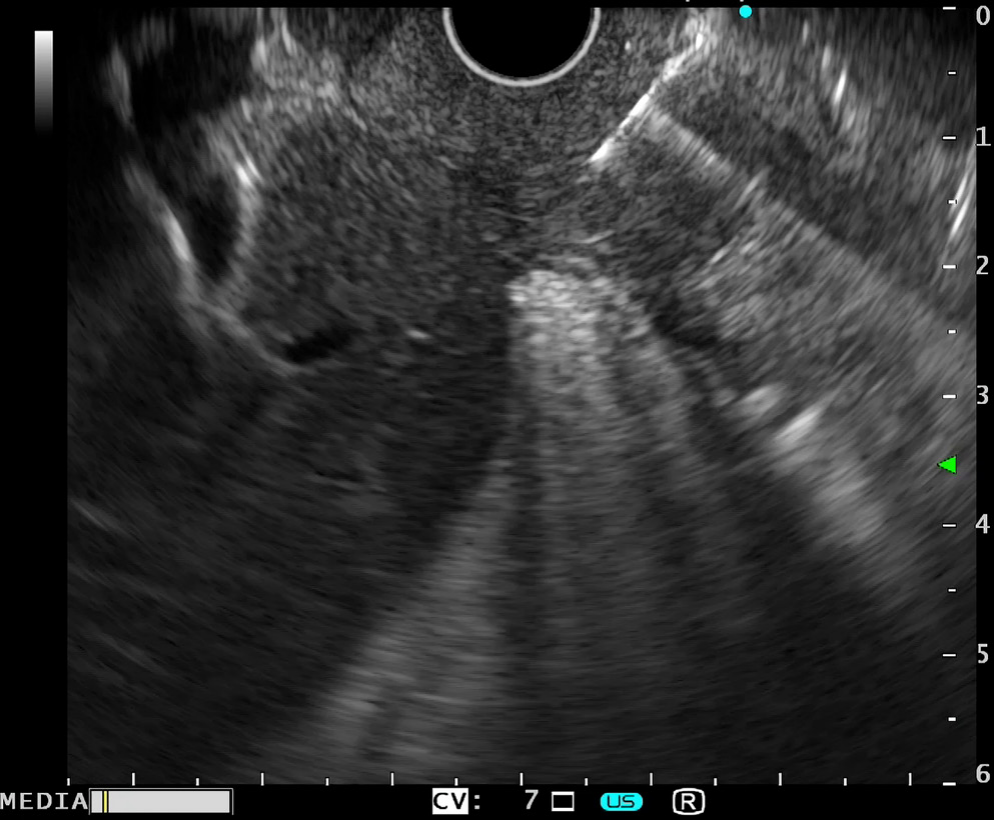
Endoscopic ultrasound fine-needle biopsy of the thickened duodenal wall.
